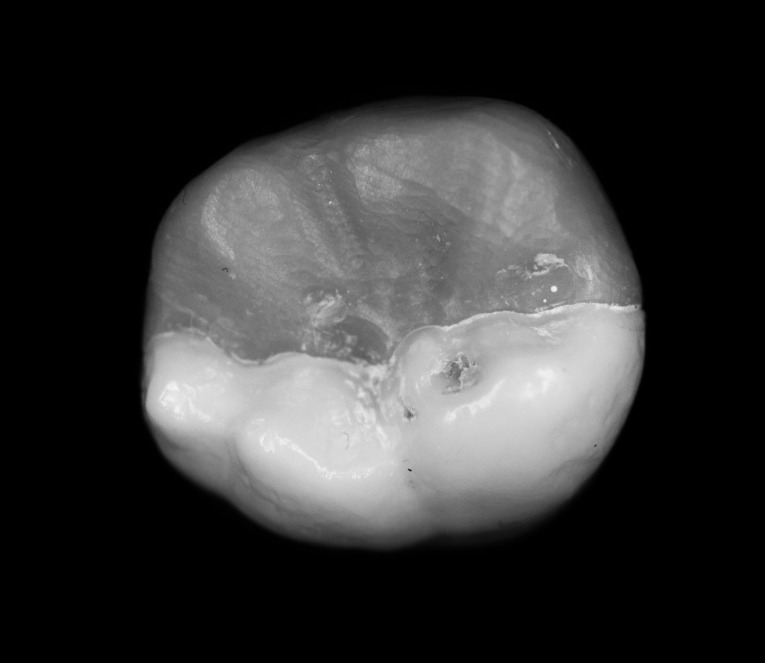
This preliminary study had a small sample size, and the assessment was limited, as the restorations were only assessed through (1) photographs, (2) radiographs, and a (3) clinical post-cementation checklist on stand-alone extracted teeth. Further evaluations are required with larger sample sizes, adjacent teeth, antagonists, other materials, physical testing, and a clinical study.
